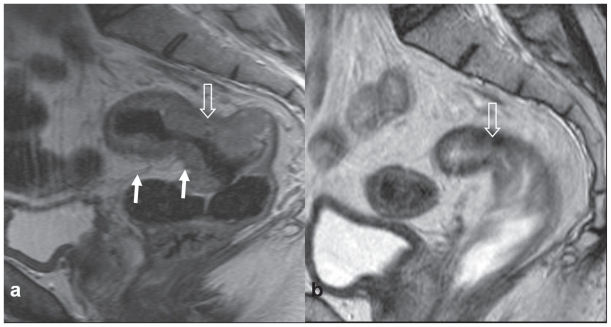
Patient 1: Sagittal T2 weighted fast spin echo (TR3900 TE 120) MRI scans of a) pre-chemoradiotherapy, showing a large rectal tumour (open arrow) almost entirely above the peritoneal reflection (arrow), and b) marked tumour regression and downsizing 4 weeks post-chemoradiotherapy, with areas of low signal intensity indicating fibrosis (open arrow).
